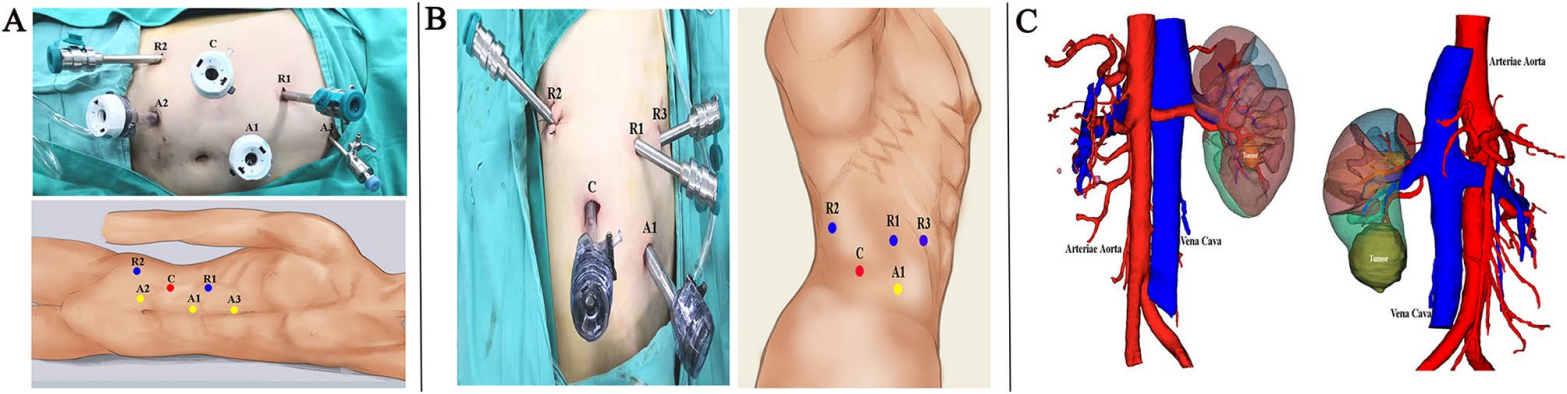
Patient position, port placement, and preoperative three-dimensional (3D) imaging. A Via transperitoneal approach (right side): C, trocar for camera; R1, robotic trocar for 1st arm; R2, robotic trocar for 2nd arm; A1, 12-mm trocar for assistant; A2, 12-mm trocar for assistant; A3, 5-mm trocar for assistant. B Via retroperitoneal approach (right side): C, trocar for camera; R1, robotic trocar for 1st arm; R2, robotic trocar for 2nd arm; R3, robotic trocar for 3rd arm; A1, 12-mm trocar for assistant. C 3D reconstruction from CT imaging showing the kidney mass, segmental arteries of the kidney, and renal calyces
